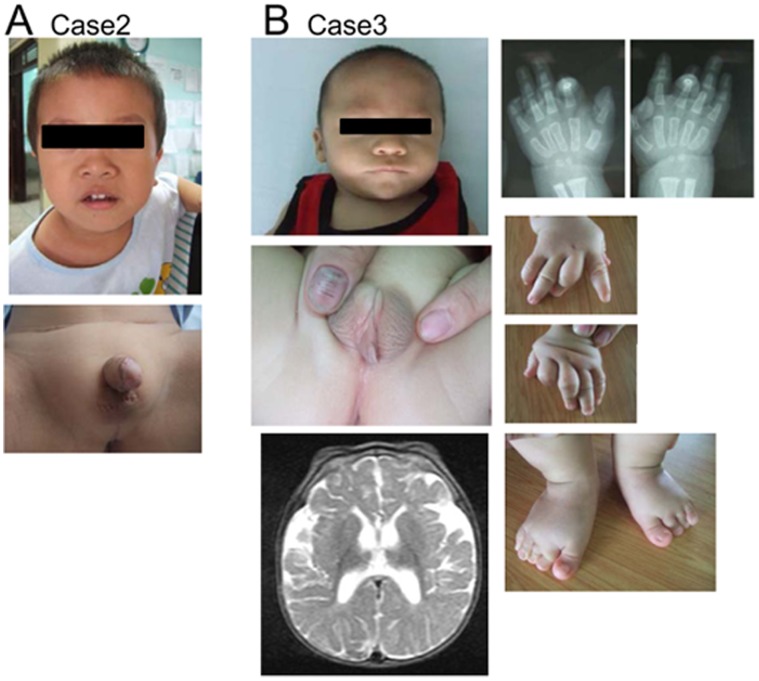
Clinical features of cases 2 and 3. A. Clinical findings of case 2 at 4.5 years of age. Images of the craniofacial region and external genitalia (after surgical intervention) are shown. B. Clinical findings of case 3 at 11 months of age. Multiple facial dysmorphisms and limb anomalies including syndactyly and camptodactyly are shown. Brain magnetic resonance imaging indicates delayed myelination, hypogenesis of the corpus callosum and prominent ventricular and CSF spaces. The parents of cases 2 and 3 have given written informed consent, as outlined in the PLOS consent form, to publication of the photographs of the patients.
